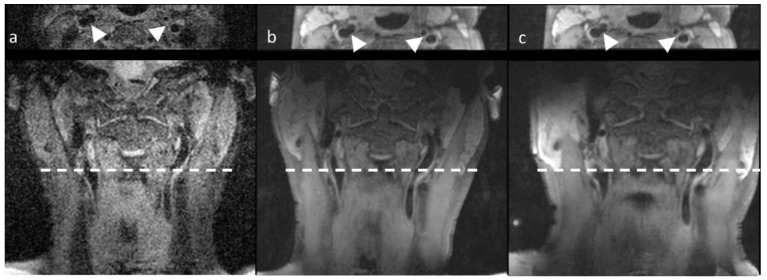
3D T1-weighted SPACE transversal and coronal views acquired with the three configurations of the carotid bifurcation. The upper panel shows transversal images, while the lower panels show coronal images. (a) No surface coil, (b) MRI-only coil, and (c) PET/MRI coil. White arrows in the transversal images indicate the left and right carotid arteries.
